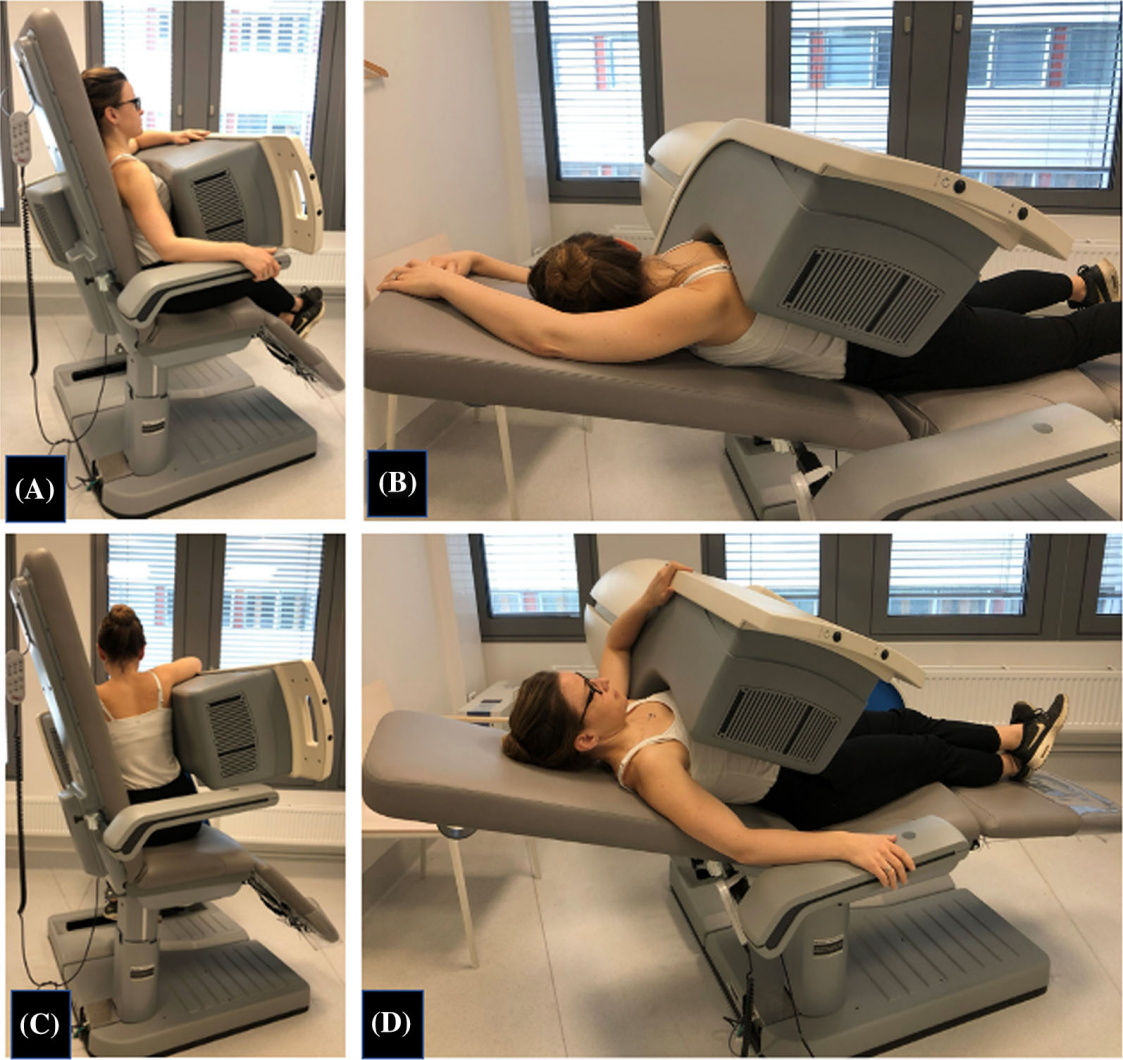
In the D-SPECT patients with normal position of the heart (levocardia) are usually examined in both supine and upright position to enable assessment of attenuation: A upright position B Supine position. In the patient with dextrocardia the position in the chair had to be adjusted so the heart could correctly be centered. C Upright positioning at approximately 70° chair angle the heart could be centered within the positioning ellipse. The patient can still sit comfortably by supporting the arms on top of the detector and leaning towards the backrest. D By lowering the backrest at approximately 20° chair angle the patient can be in a prone position, and with a tight positioning of the detector arm around the patients back the left ventricle can be centered within the positioning ellipse
